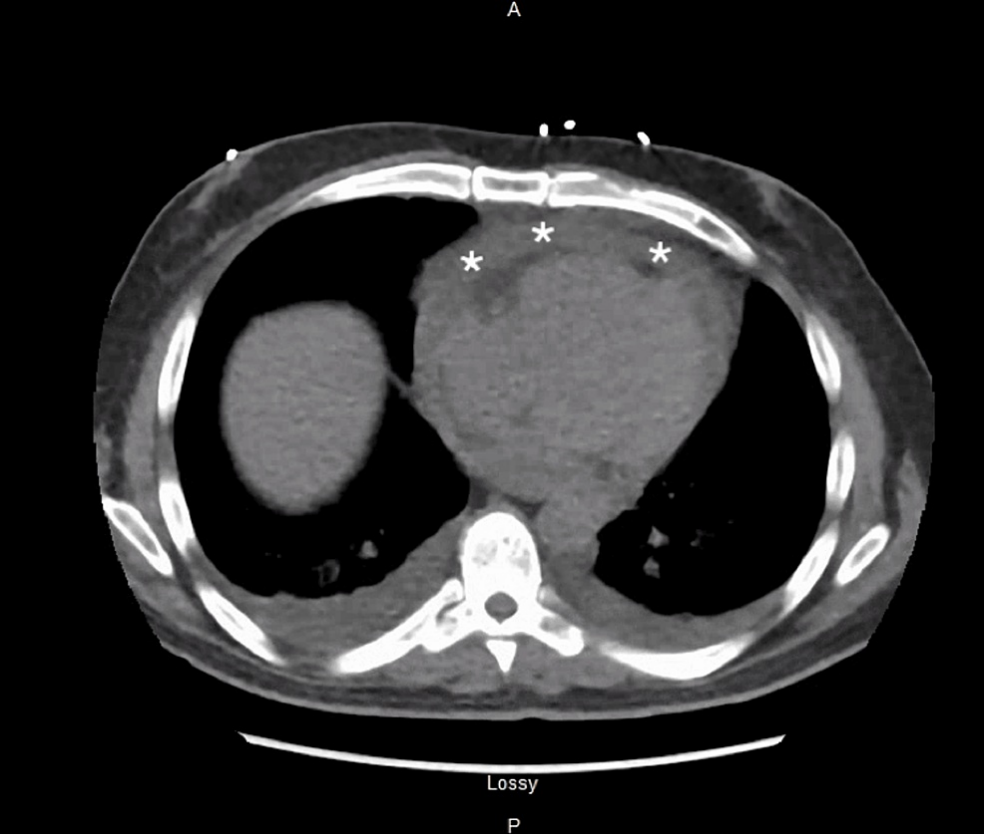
CT scan showing pericardial effusion (asterisks)
